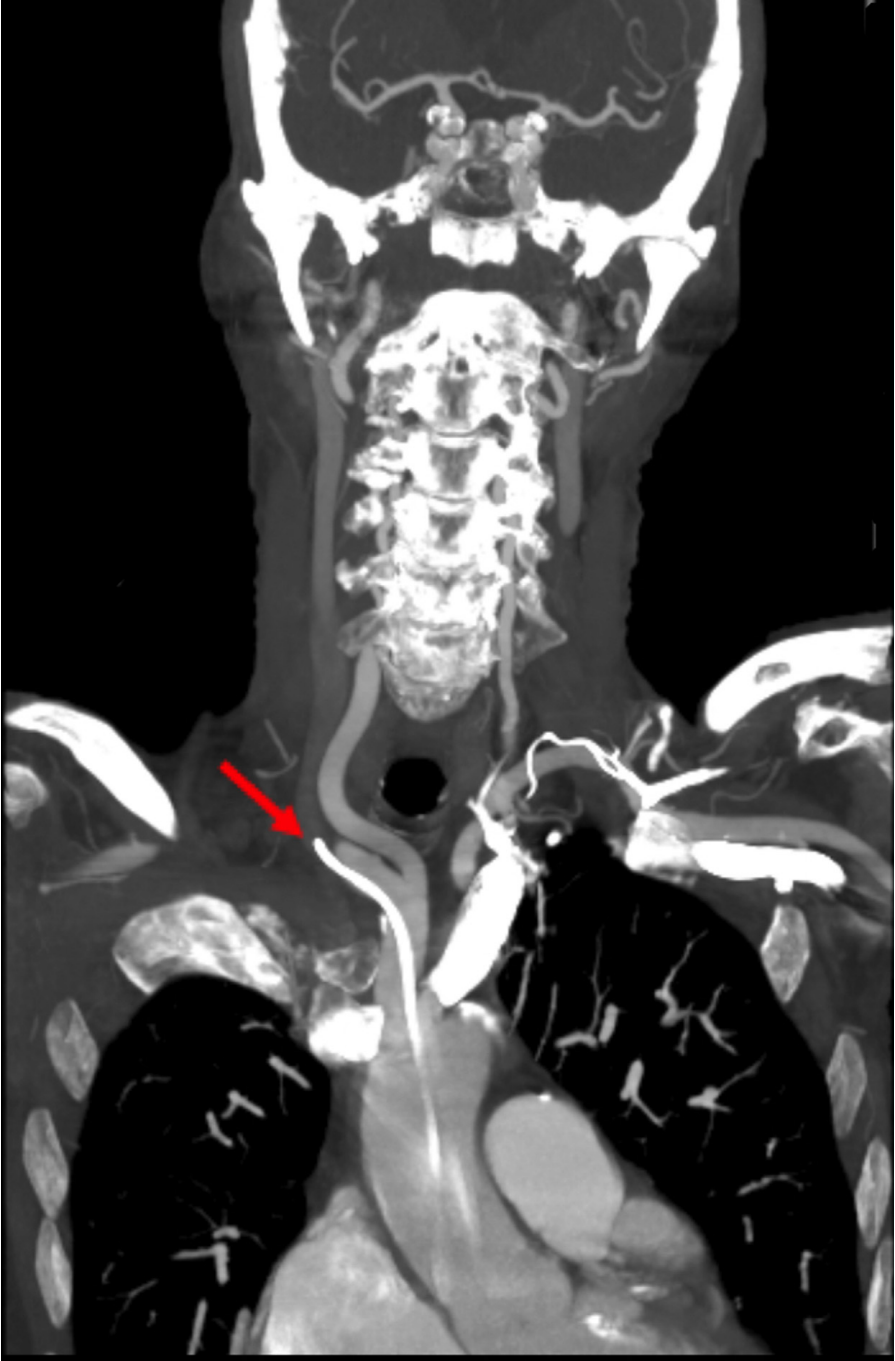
CT angiogram (coronal view). Red arrows showing the malposition Port‐A‐Cath.
